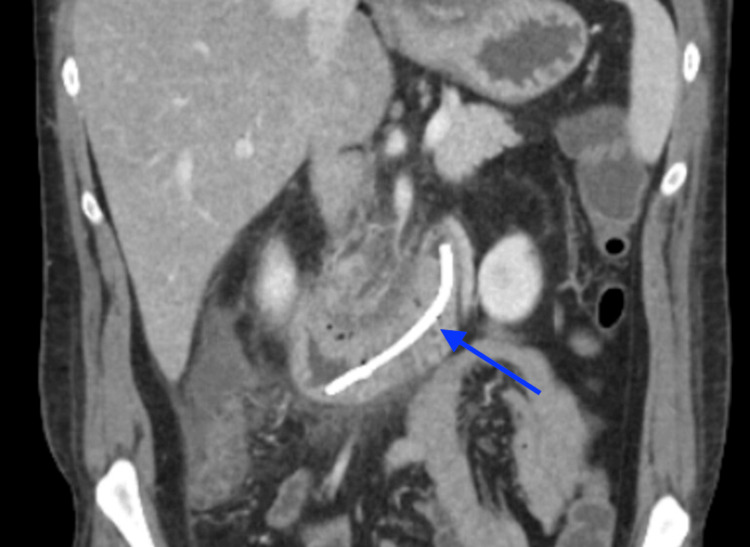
CT in coronal view, blue arrow pointing to a migrated pancreatic duct stent located within the duodenum
